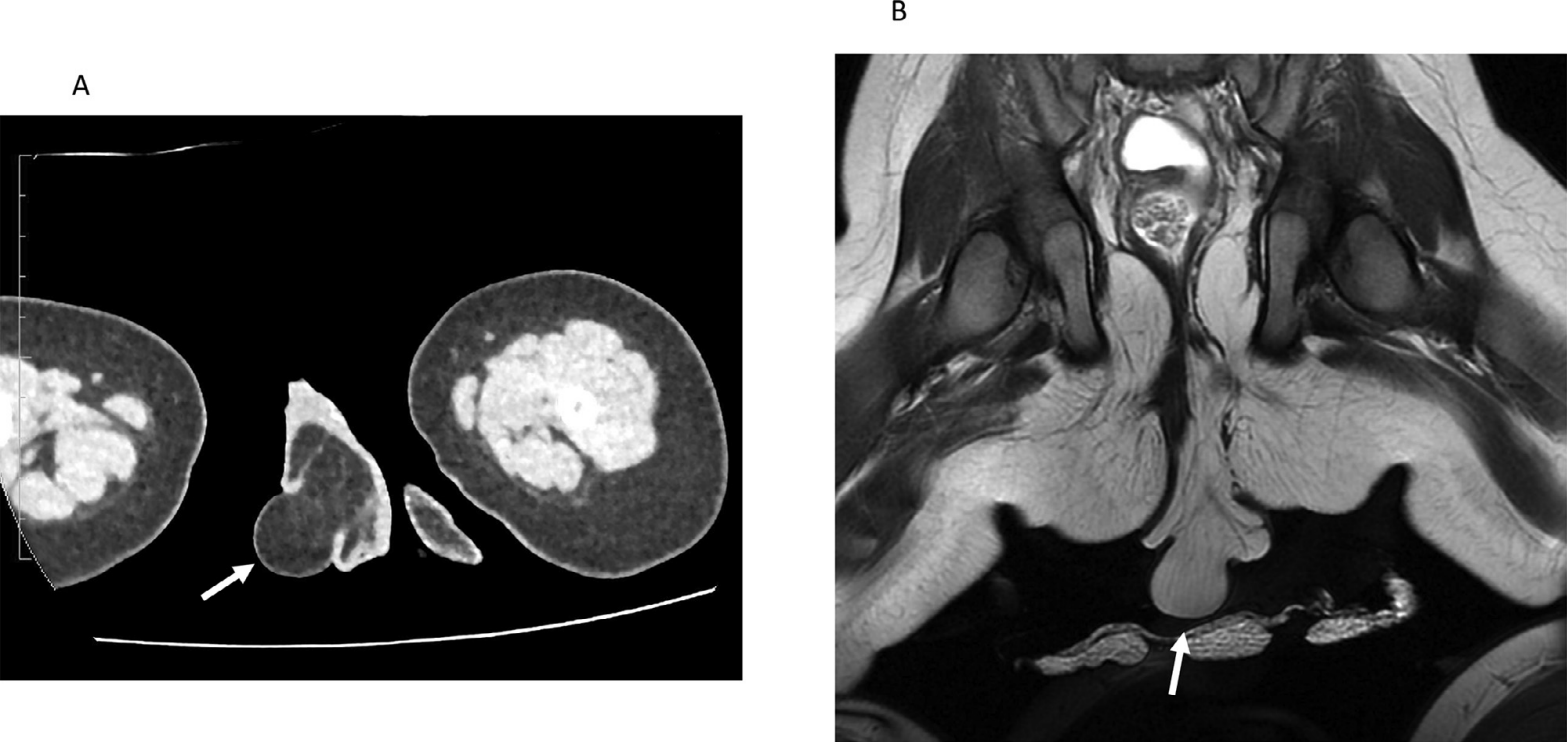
Postnatal images. (A) A computed tomography image shows an accessory scrotum with perineal lipoma. (B) A postnatal magnetic resonance image shows an accessory scrotum with perineal lipoma (arrow).
